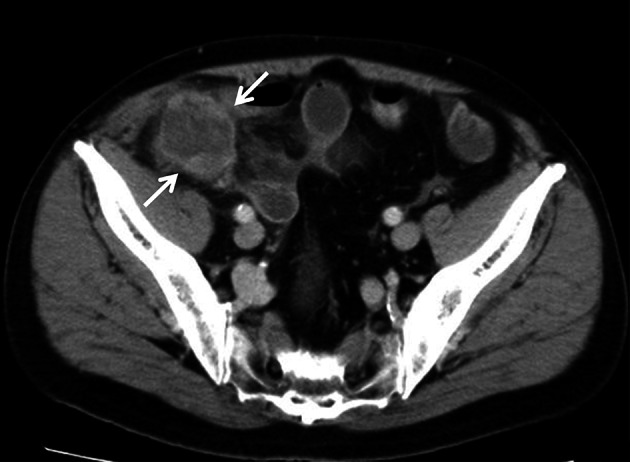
Abdominal CT. Abdominal CT revealed a mass with peripheral enhancement approximately 5 cm in diameter (arrow)
